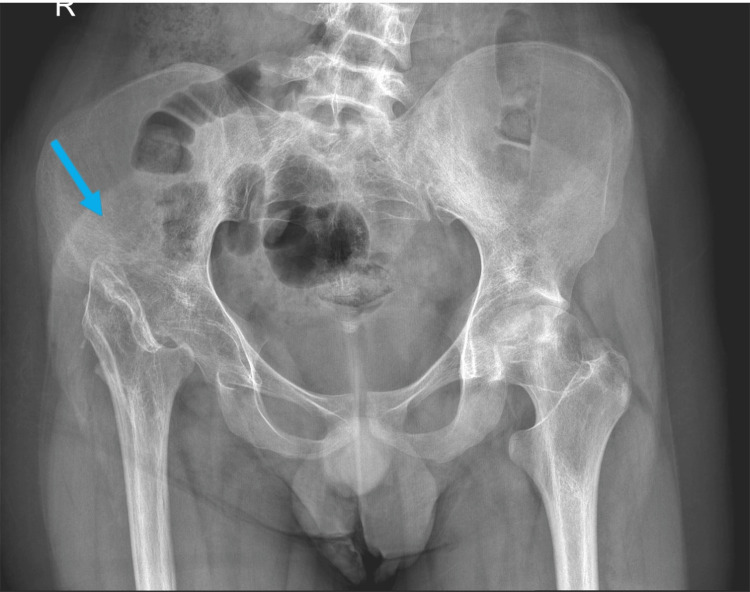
Pre-THR X-ray image of the pelvis THR: Total Hip Arthroplasty
